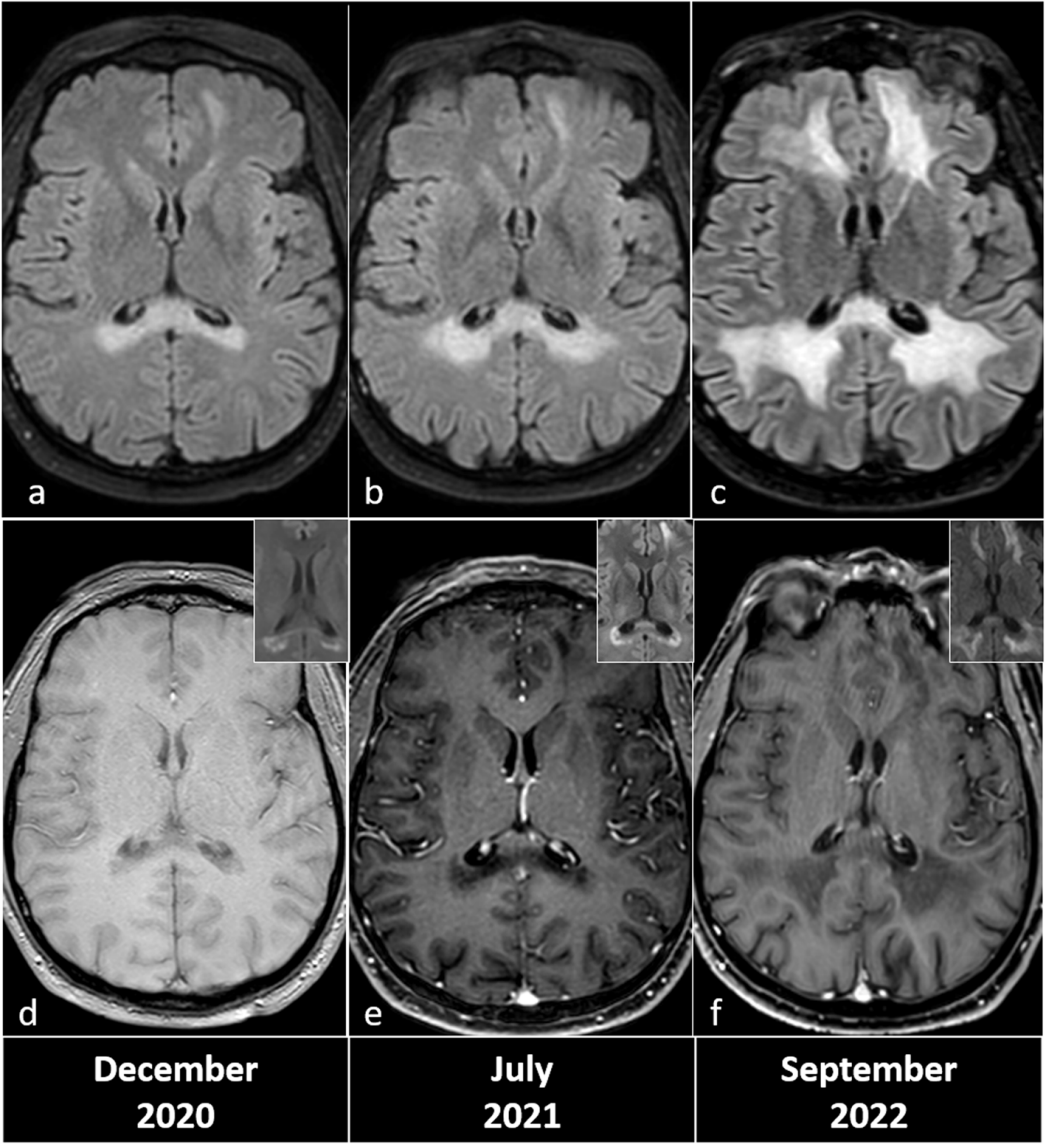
Progression of cerebral white matter abnormalities in a 38-year-old patient affected with adrenoleukodystrophy. Axial fluid-attenuated inversion recovery (FLAIR) images show a slight increase in lesions from September 2020 (a) to July 2021 (b) and an abrupt progression in September 2022 (c). A three-dimensional T1-weighted gradient-echo sequence failed to demonstrate enhancement in July 2021 (e) and September 2022 (f), while on September 2020, magnetic resonance imaging was performed without contrast injection (d, axial T1-weighted image). High *b*-value on diffusion-weighted imaging constantly shows a fringe of potential disease activity at the edge of lesions (insets in d, e, and f)
